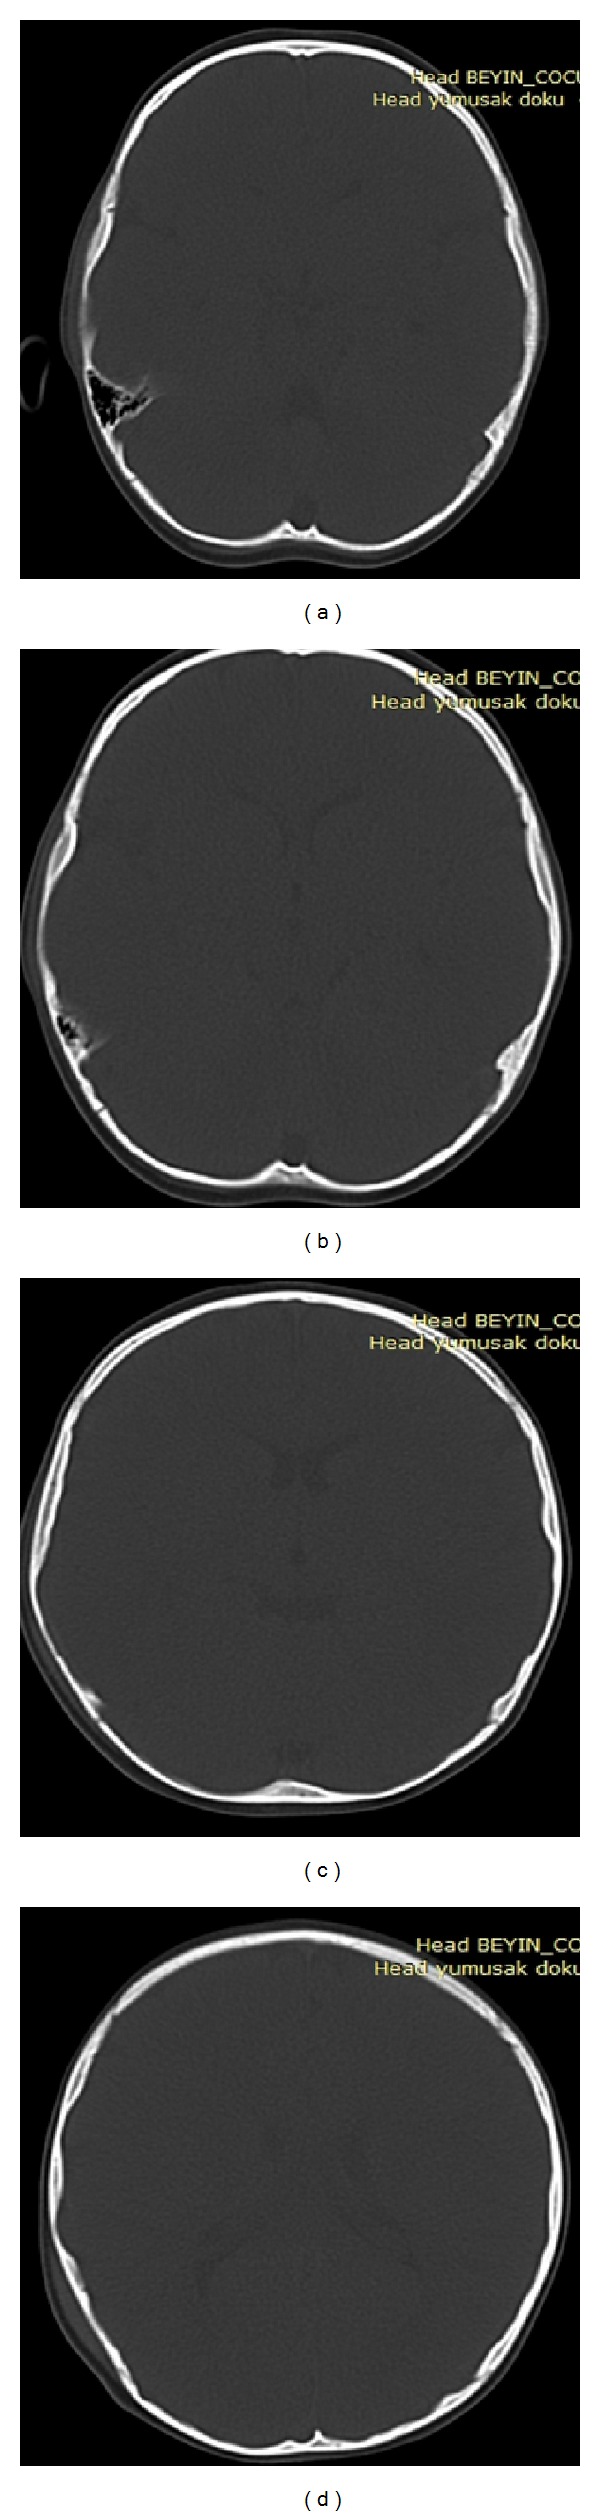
Axial CT image at different levels with no fracture. (a) Level of mastoid air cells. (b) Level of temporal region. (c) Level of lateral ventricles. (d) Level of centrum semiovale (there is a subgaleal hematoma indicated with white arrow).
